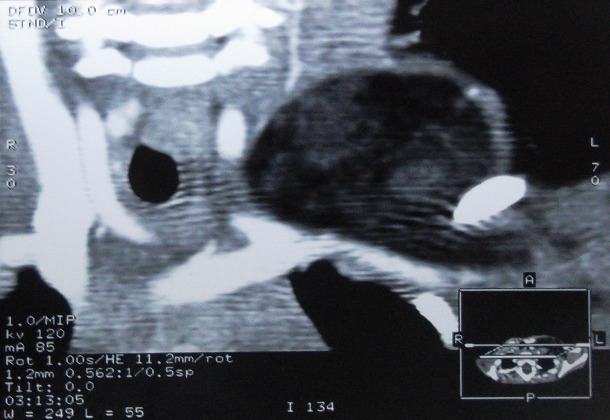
Cervical CT scann view of a heterogeneous, well-defined lesion with hypervascularity
